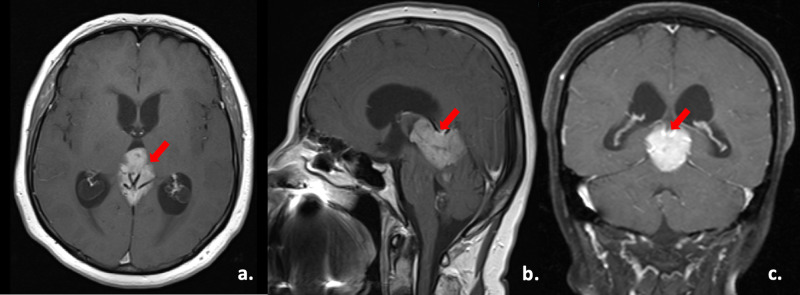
Magnetic resonance images of the mass a, b and c: Axial, sagittal, and coronal contrast-enhanced magnetic resonance imaging (MRI) scans of the mass originating from the pineal region (red arrows), respectively.
